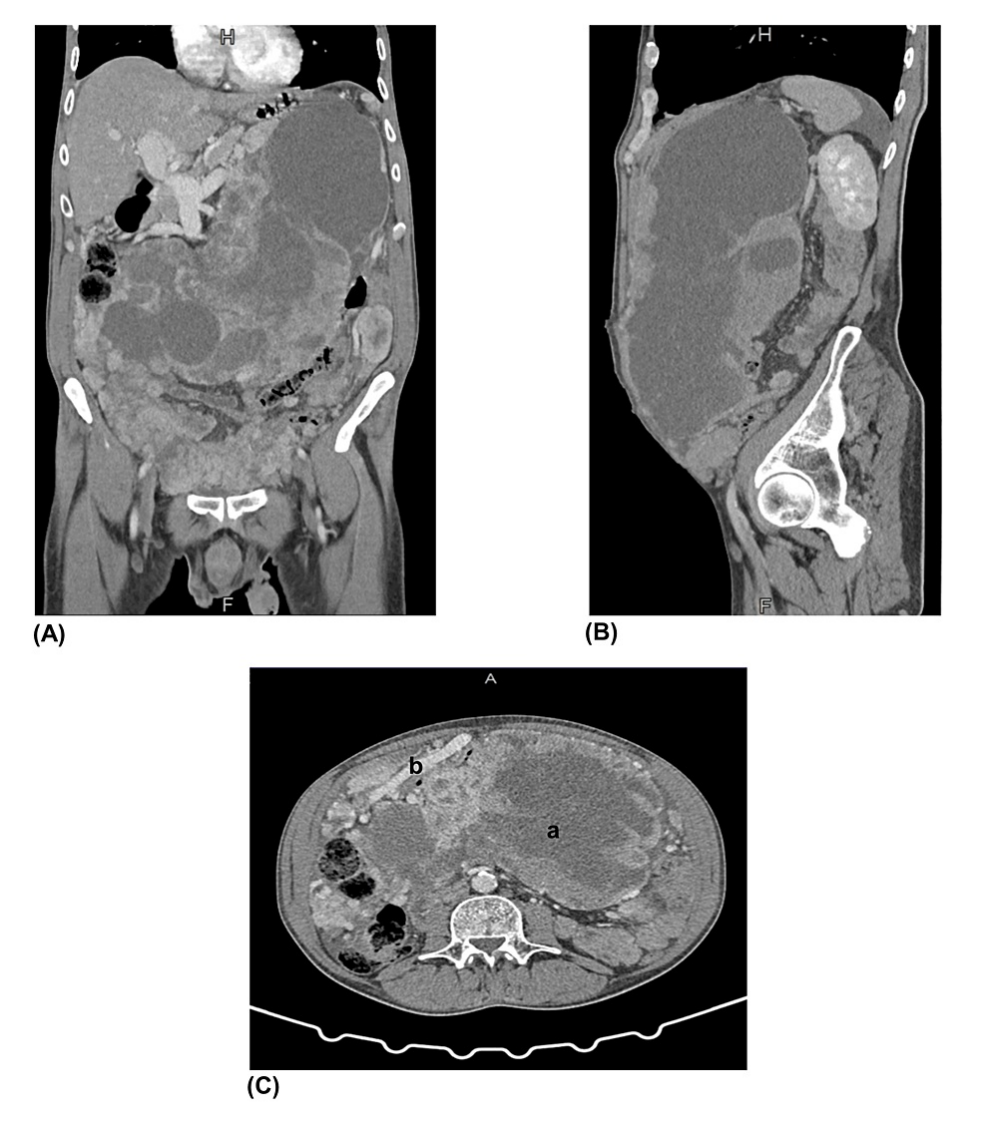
Abdominopelvic contrast-enhanced CT scan. (A) coronal plane, arterial phase; (B) sagittal plane, portal phase; (C) horizontal plane, arterial phase: a - areas of necrotic component with no enhancement; b - dilatation of a vascular structure next to the heterogenous tumor. CT, computed tomography
